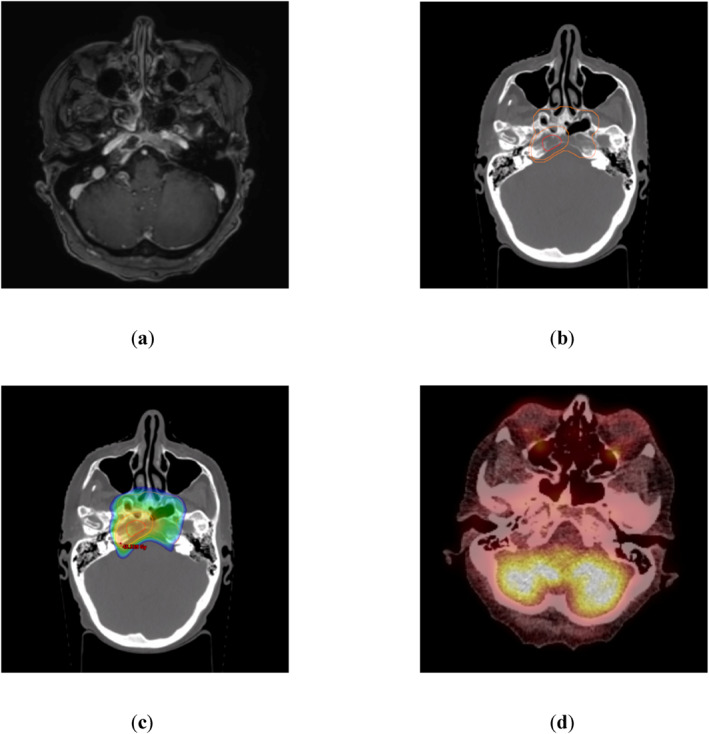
Bridging radiotherapy (RT) for a 71‐year‐old woman presenting with serological progression and abducens paresis due to a lesion in the clivus under bridging therapy with isatuximab, pomalidomide and dexamethasone prior to chimeric antigen receptor T‐cell (CAR‐T) therapy. Interdisciplinary tumor board voted for modified bridging strategy with talquetamab and concomitant local RT of clival lesion. No more lesions were detected in low‐dose computer tomography (CT) (a) Magnetic resonance imaging (MRI) showing a T1‐contrast‐enhancing lesion in the right clivus (b) Planning‐CT indicating the MRI‐based Gross Tumor Volume (red) and Planning Target Volume (orange). Cone‐down‐technique was used with a sequential boost. (c) 95%‐isodose as color‐wash delineation based on a 6 MV intensity‐modulated‐radiotherapy‐plan using volumetric‐arc‐technique [prescribed dose 36 Gy in fractions of 2 Gy with sequential boost to 44 Gy (orange contour)] (d) Positron emission tomography‐CT scan 30 days after completion of RT and before planned CAR‐T therapy revealing no metabolic activity. At the most recent follow‐up, 12 months after CAR‐T therapy, a sustained complete remission was documented.
